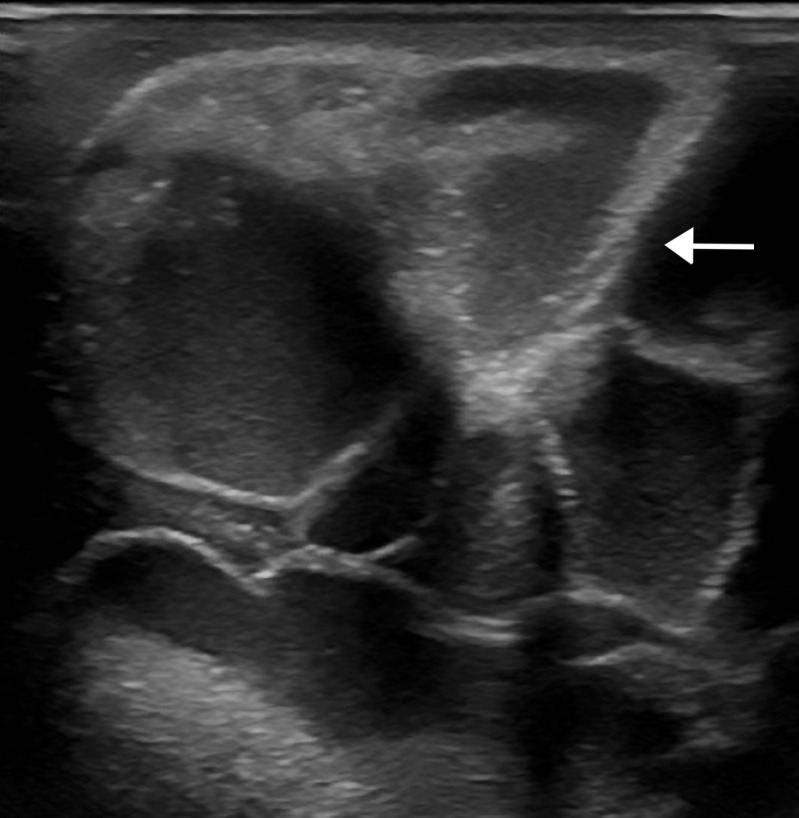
Right forearm ultrasound Grayscale ultrasound image demonstrating large, echogenic, lobulated calcifications (arrow) in the dorsal proximal right forearm.
